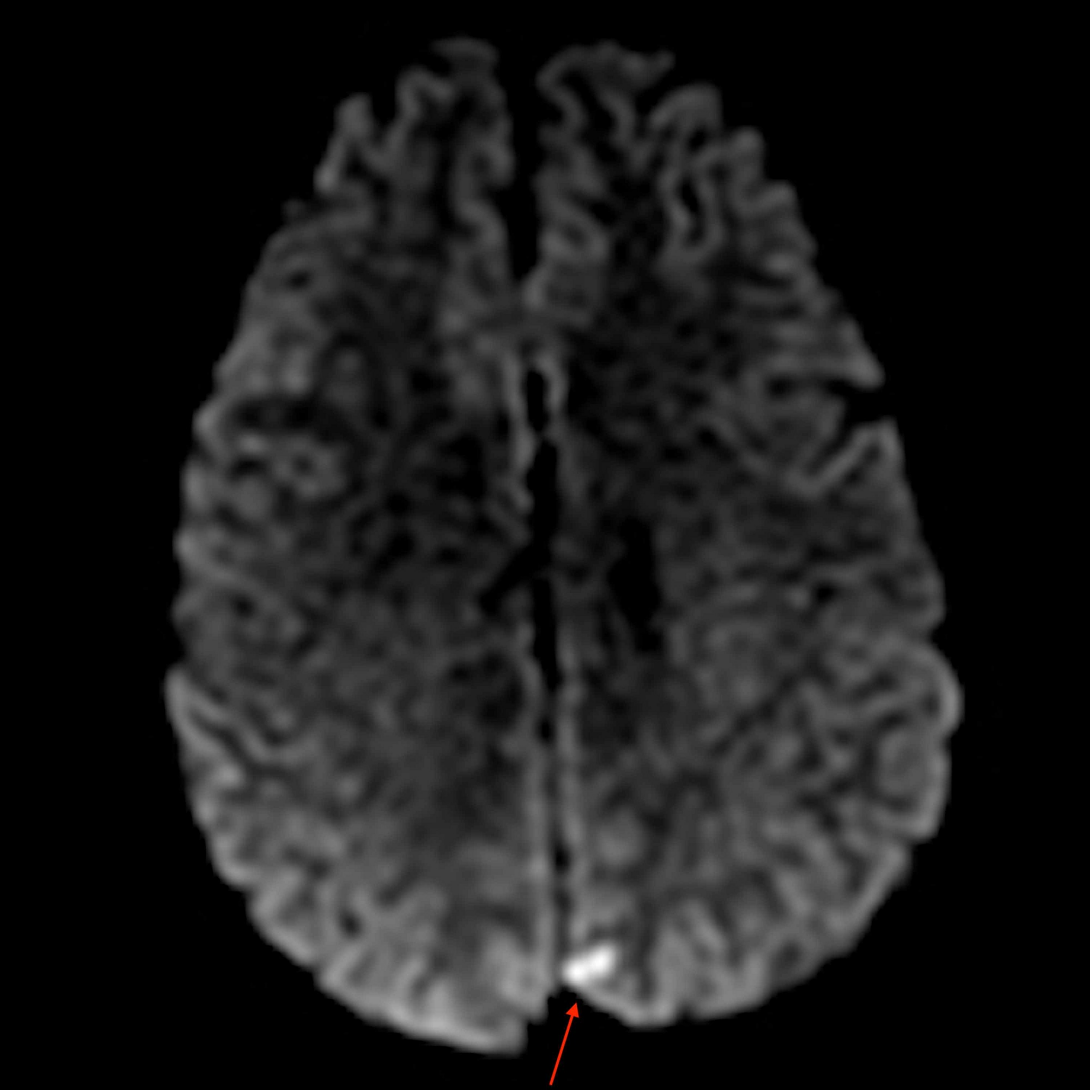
Diffusion-weighted MRI shows a small focus of diffusion restriction in the left occipital cortex compatible with an acute small vessel embolic infarct.
